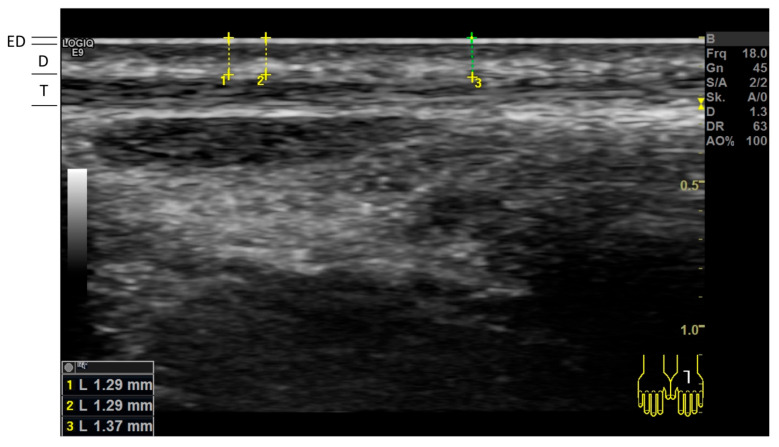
High-frequency ultrasonography of the dorsal left hand: epidermal (ED) layer, dermal (D) layer, and extensor tendon (T). Three measurements of the cutis thickness (ED and D layer combined) were performed for each image in areas with best discriminability of the border between the dermis and hypodermal structures.
